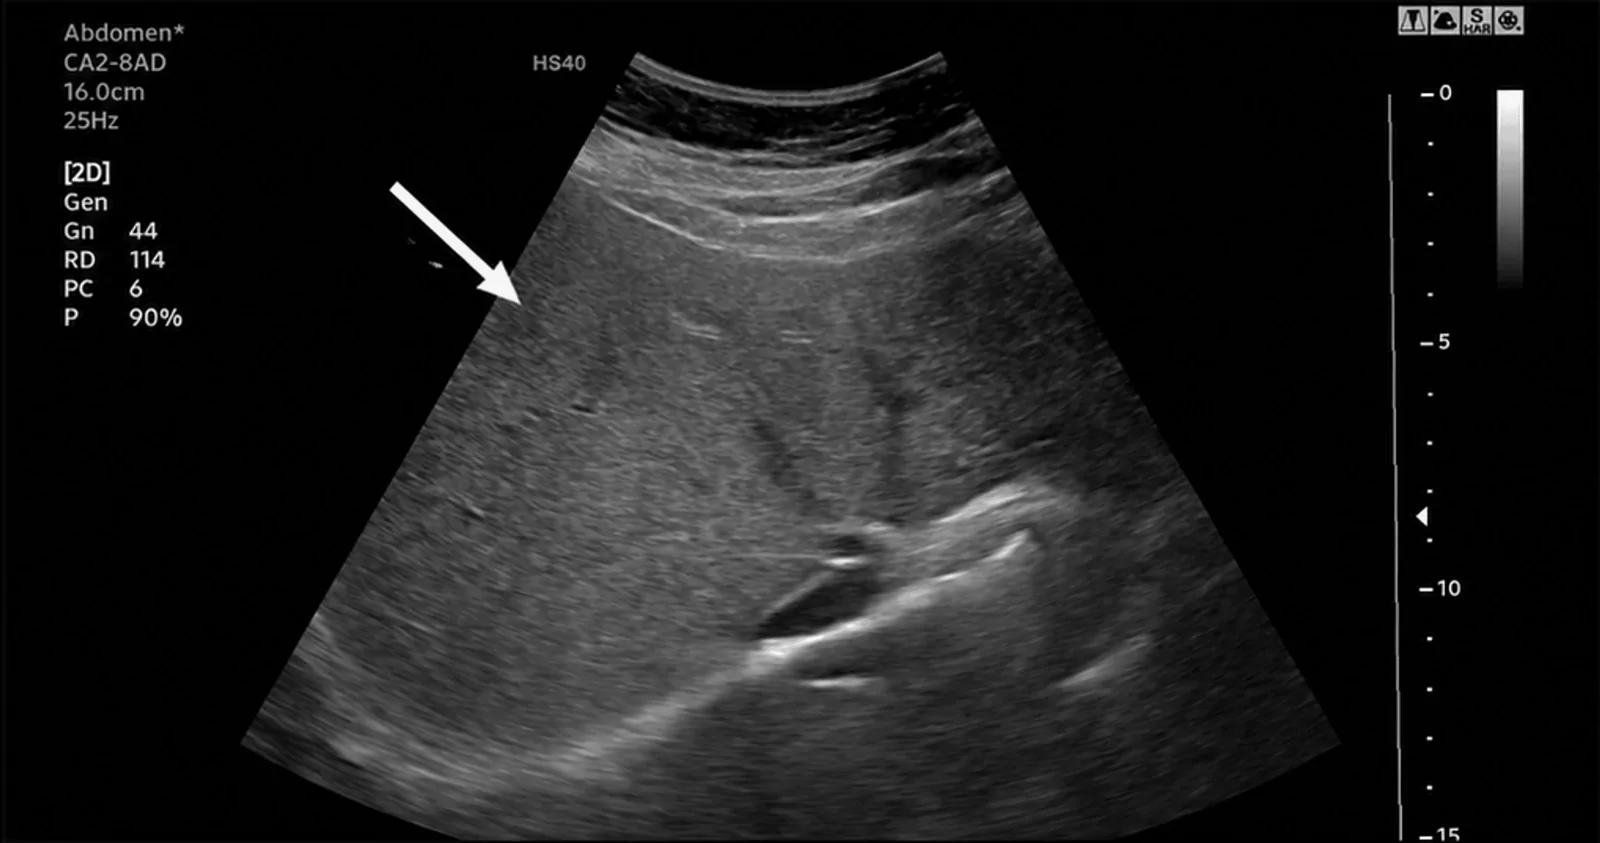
Abdominal ultrasound demonstrating hepatic steatosis Abdominal ultrasound obtained from the patient presented in this case. The arrow indicates the hepatic parenchyma demonstrating diffuse increased echogenicity consistent with hepatic steatosis
